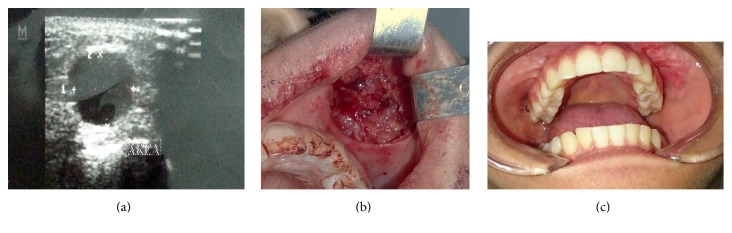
(a) Ultrasound examination showing the presence of a hypoechoic tumoral mass. (b) Transoperative view. (c) Follow-up at 8 months.
